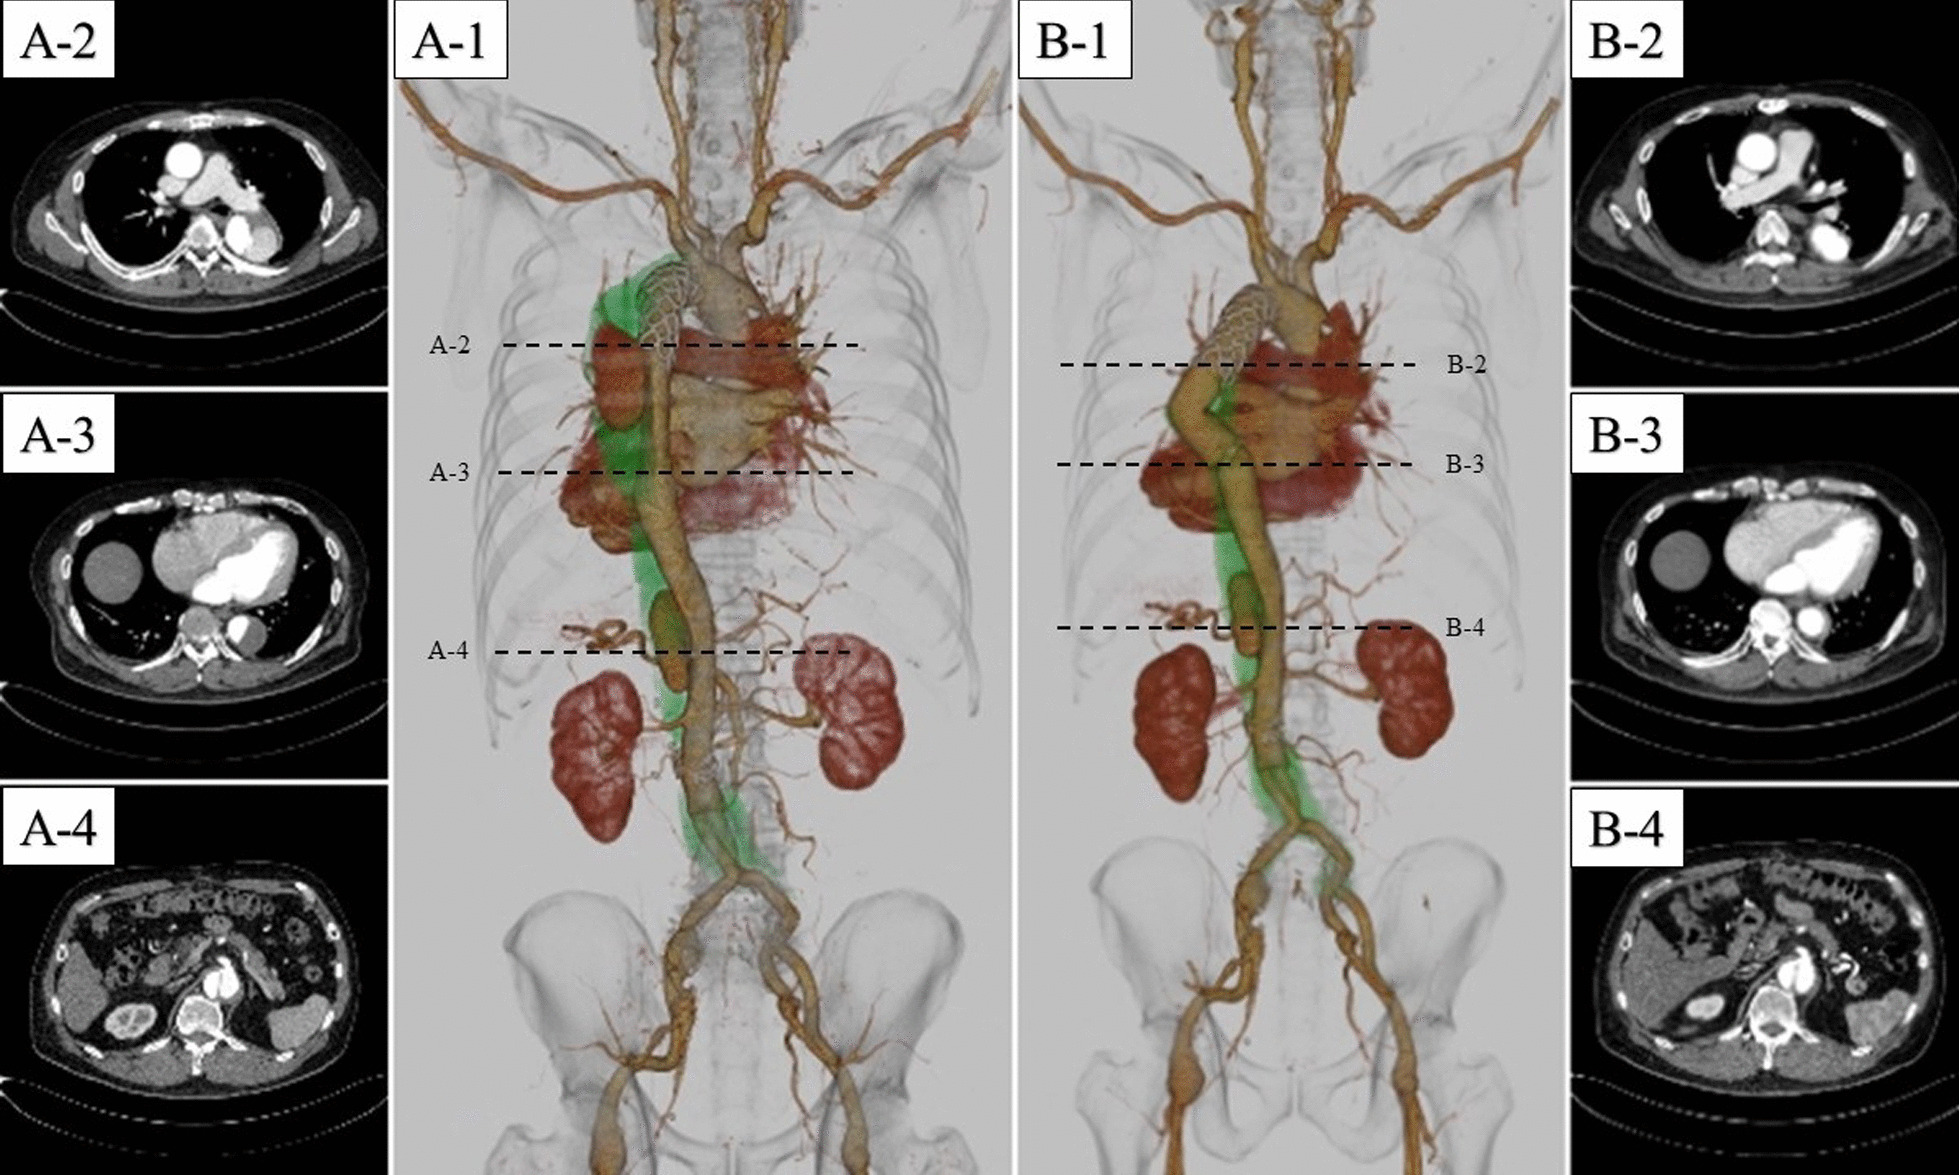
Preoperative (A1–4) and postoperative (B1–4) computed tomography. A2 shows the stent graft-induced new entry lesion. B2 shows that the entry lesion was successfully excluded
